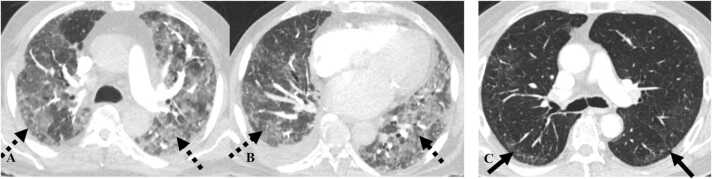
Immune-mediated pneumonitis. A&B. Contrast-enhanced axial chest CT images in a 63-year-old man with non-small-cell lung cancer on pembrolizumab show bilateral ground glass opacities with mild traction bronchiectasis (dashed black arrows) representing acute interstitial pneumonia or acute respiratory distress pattern of pneumonitis. C. Contrast-enhanced axial chest CT image in a 78-year-old man with melanoma on pembrolizumab shows peripheral reticulations with subpleural sparing (black arrows) consistent with NSIP pattern of pneumonitis.
